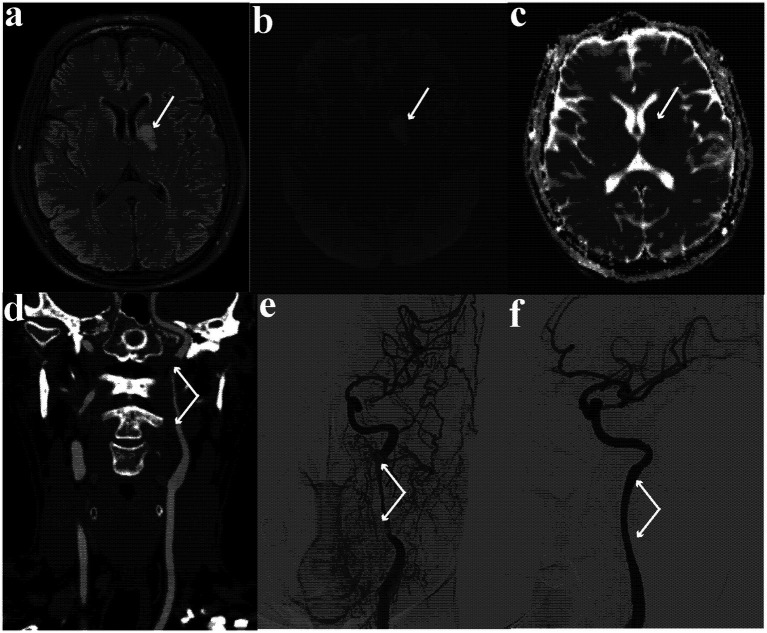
(a) Hyperintense oval lesion at left basal ganglia and inner capsule on fluid-attenuated inversion recovery image; (b) hyperintense oval lesion on diffusion-weighted imaging; (c) reduced apparent diffusion coefficient at the lesion; (d) near occlusion at the upper cervical segment of internal carotid artery and wave-like tunica intima of proximal carotid artery on computed tomography angiogram; (e) carotid dissection with severe stenosis on digital subtraction angiography; (f) repaired internal carotid artery and patent left ICA lumen.
